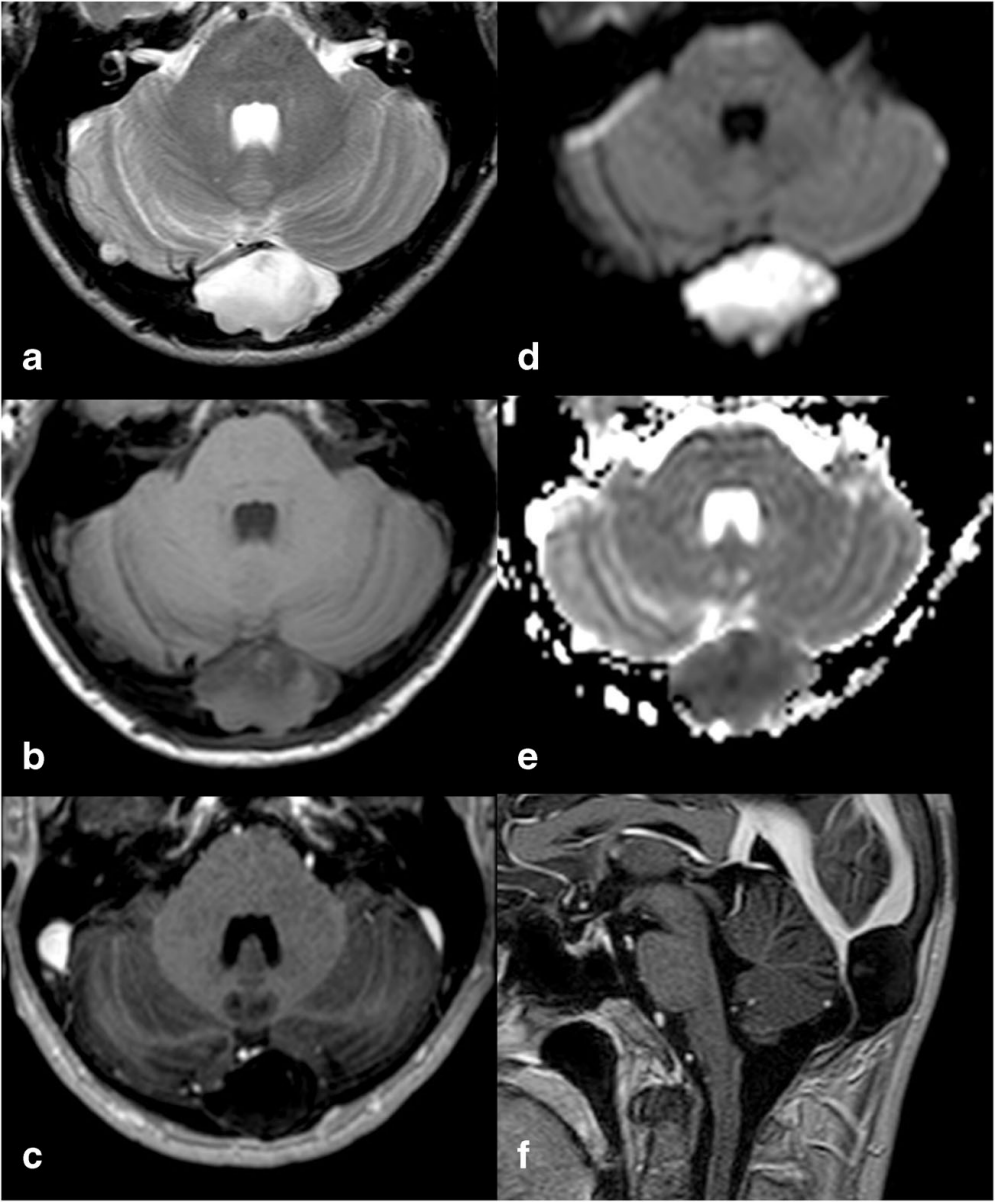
IEC. On MR images of the same patient shown in Fig. 3, the lesion appears slightly inhomogeneous, mostly hyperintense on T2w (a) and hypointense on T1w (b) images, without contrast enhancement (c), showing diffusion restriction on *b* 1000 diffusion-weighted imaging (DWI) and the apparent diffusion coefficient (ADC) map (d, e). It causes compression on the confluence of sinuses (sagittal contrast-enhanced T1w image, f). As a consequence, the patient presented with intracranial hypertension symptoms
